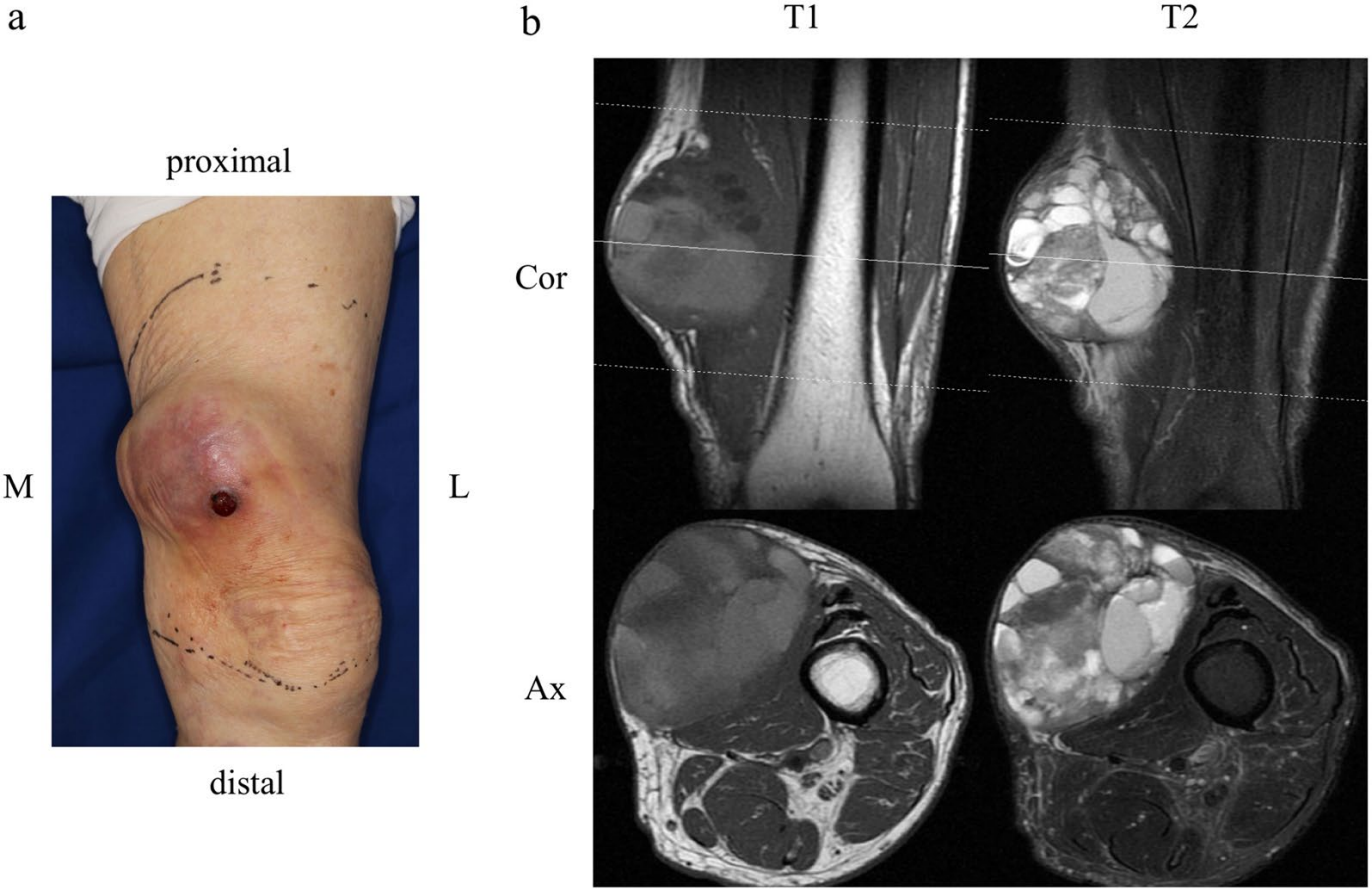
Preoperative physical condition a and magnetic resonance imaging scans b of the femur. a Left medial femur with a tumour mass. b Soft tissue mass with internal heterogeneity, cystic degeneration, and fluid–fluid level. *M* medial, *L* lateral, *T1* T1-weighted images, *T2* T2-weighted images, *Cor* coronal, *Ax* axial
